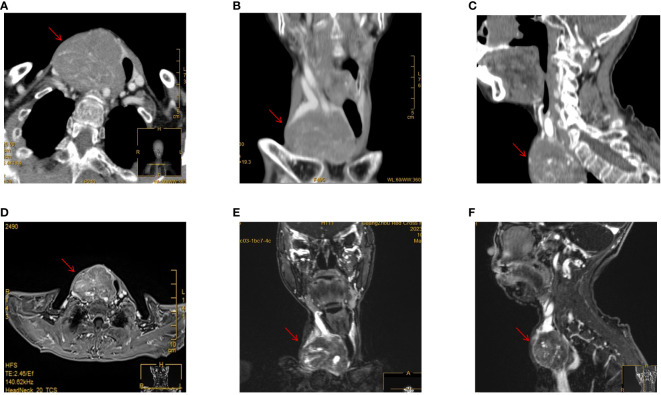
CT and MRI examinations. (A) Enhanced CT scan of the neck in horizontal position. (B) Enhanced CT scan of the neck in coronary position. (C) Enhanced CT scan of the neck in sagittal position. (D) Enhanced MRI scan of the neck in horizontal position. (E) Enhanced MRI scan of the neck in coronary position. (F) Enhanced MRI scan of the neck in sagittal position.
